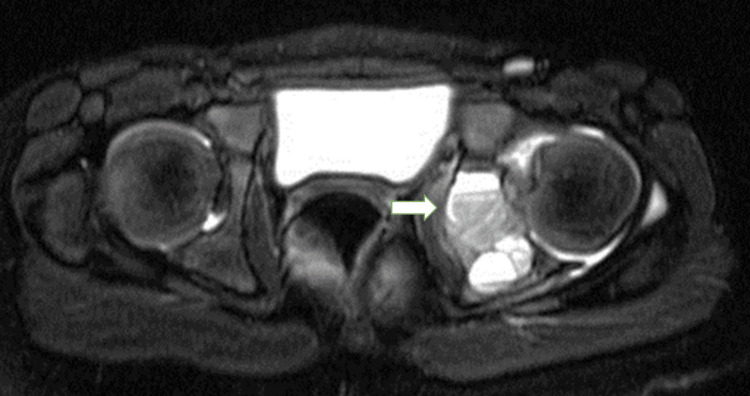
MRI axial images of an aneurysmal bone cyst of a six-year-old girl's left hip, showing septation at the posterior acetabulum with a fluid-fluid level The image belongs to the author's patient.
